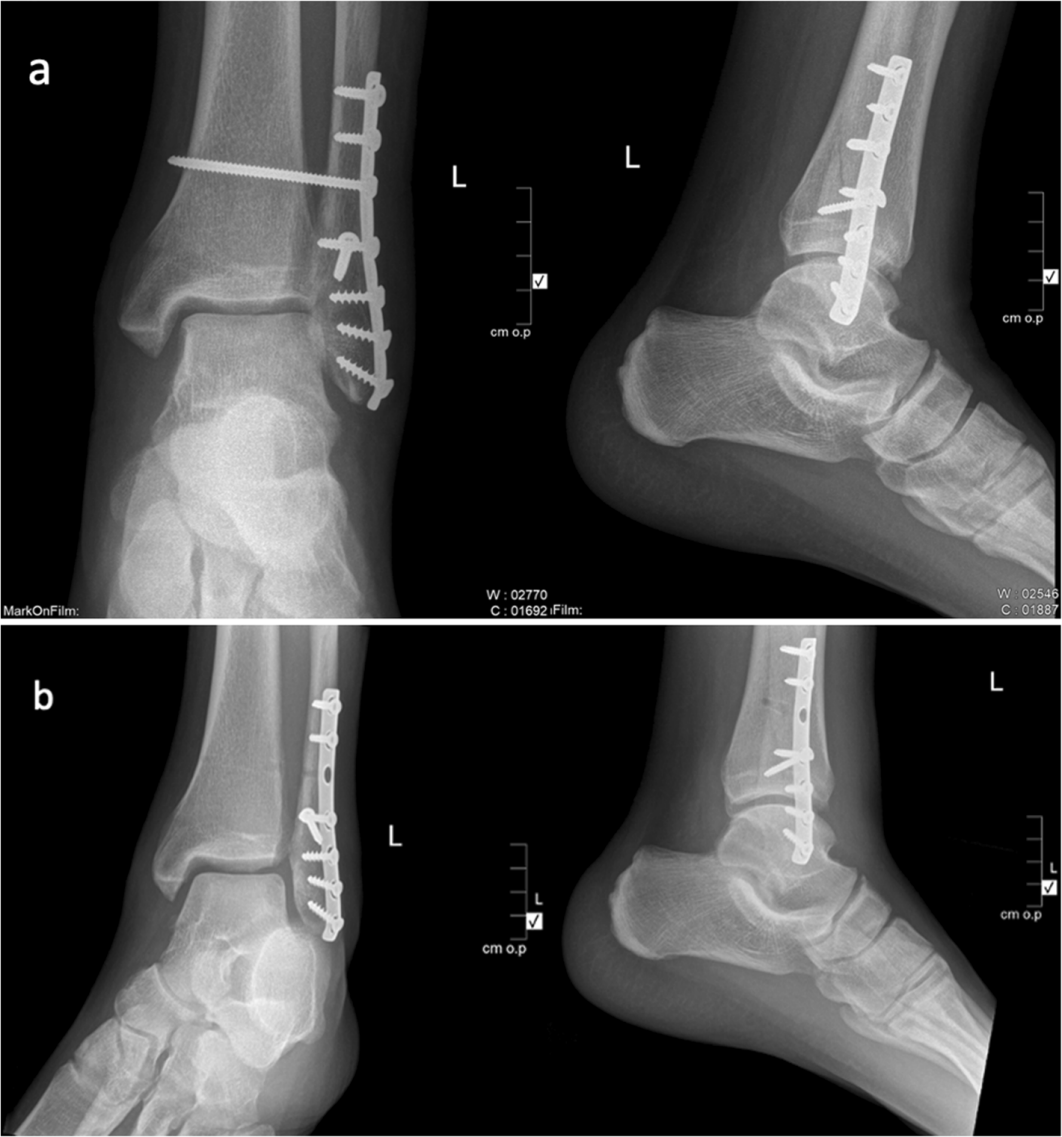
Example of recurrent diastasis after removal of syndesmotic screws. a Post-operative anteroposterior and lateral plain ankle radiographs demonstrating lateral malleolus neutralizing plate and syndesmotic screw fixation. b Post-operative anteroposterior and lateral plain ankle radiographs showing recurrent tibio-fibular diastasis after removal of the syndesmotic screw
